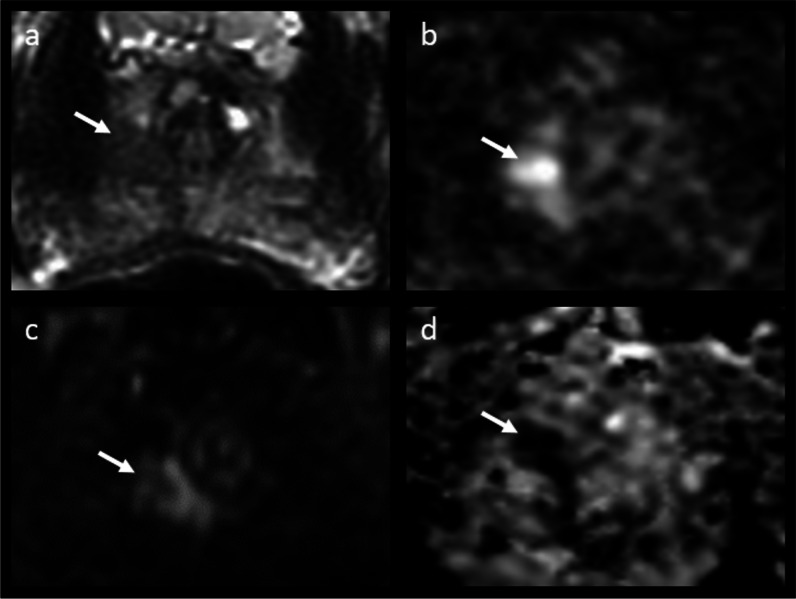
MRI of a 76-year-old patient originally reported as negative (PI-RADS 2). Elevated PSA levels (10.58 ng/mL) prompted a systematic biopsy despite MRI results, which revealed the presence of csPCa (Gleason score 3 + 4). At image retrospective review, a 4 mm hypointense lesion located at the extreme right apex was identified in the T2-weighted sequence (white arrow, a), corresponding to a focus of restricted diffusion (white arrows, b and d) with focal early enhancement on DCE (white arrow, c). The finding is in keeping with a PI-RADS 4 lesion, and the biopsy results matched the location of the MRI suspicious lesion
